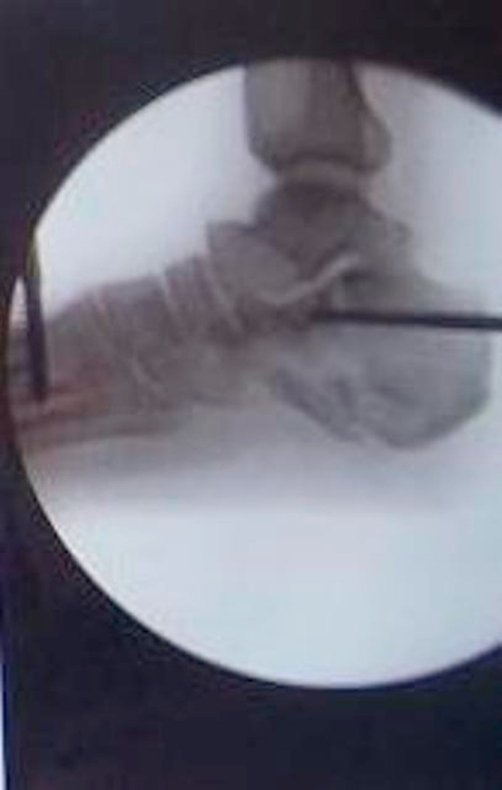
The calcaneal radiograph shows restoration of the Bohler angle.
